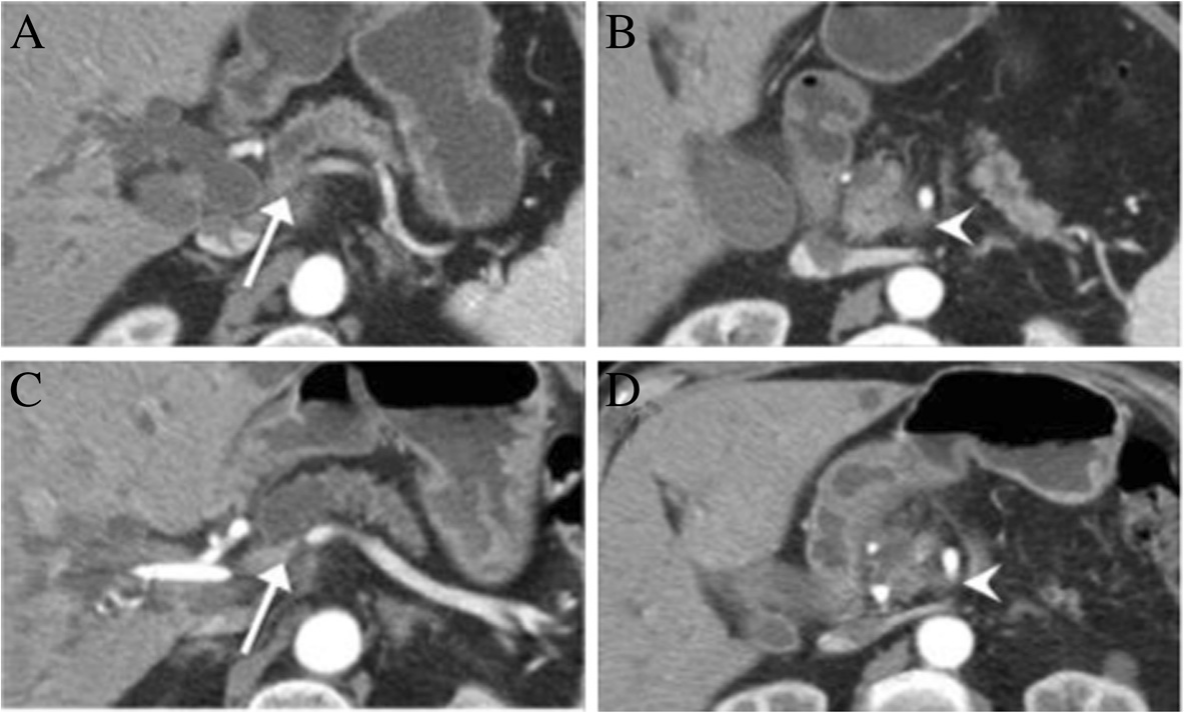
Abdominal CT images for Case 2. (A,B) Abdominal CT at the time of diagnosis, showing a pancreatic node compatible with a pancreatic tumor encasing the porto-splenic confluence (arrow) and attachment to the SMA (arrowhead); (C,D) Control CT after neoadjuvant therapy shows local disease reduction, with increased permeability of the PV (arrow) and less compromise of SMA (arrowhead).
